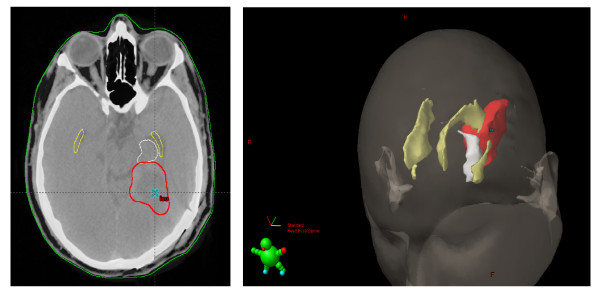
The 2-dimentional CT contouring and resulting 3-dimensional reconstruction. White: hippocampal formation. Yellow: periventricular region. Red: gross tumor volume. Reconstruction image anterosuperior view.
